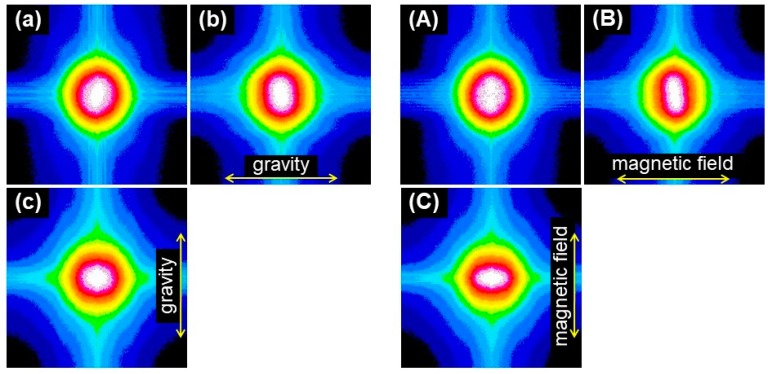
FFT images of CT photographs for magnetic elastomers with different views, (a,A) top, (b,B) side, and (c,C) front view. The direction of gravity and the magnetic field are indicated by arrows. (CI 70 wt%, DOP 70 wt%).
